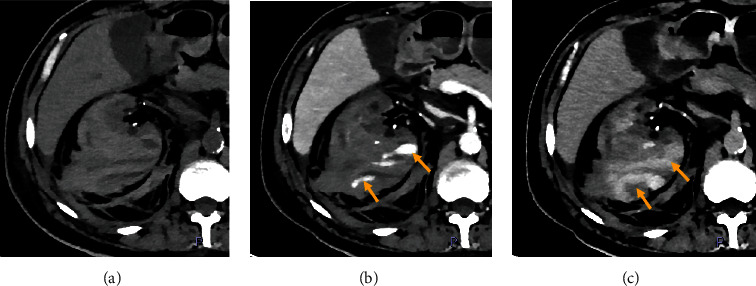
Axial nonenhancement (a), portal venous (b), and delayed phases (c) CT images show a subcapsular and perirenal hematoma on the right kidney. The portal venous phase (b) shows contrast extravasation (arrows) and changes in size, attenuation, and shape in the delayed phase (c).
